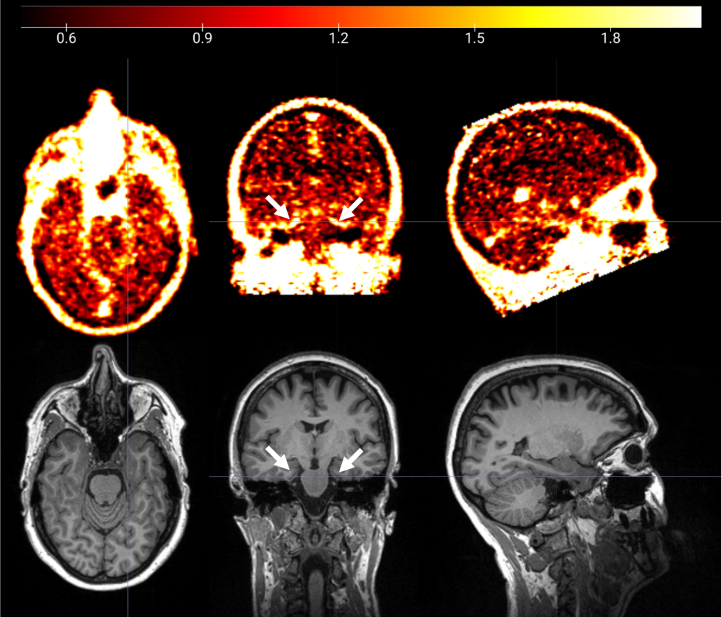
^18^F-GE180 PET SUVR image (upper panel) and T1 structural MRI image (lower panel) from a cognitively impaired participant. Elevated ^18^F-GE180 uptake following the contour of entorhinal cortex and parahippocampal gyrus (pointed by white arrows) was observed.
